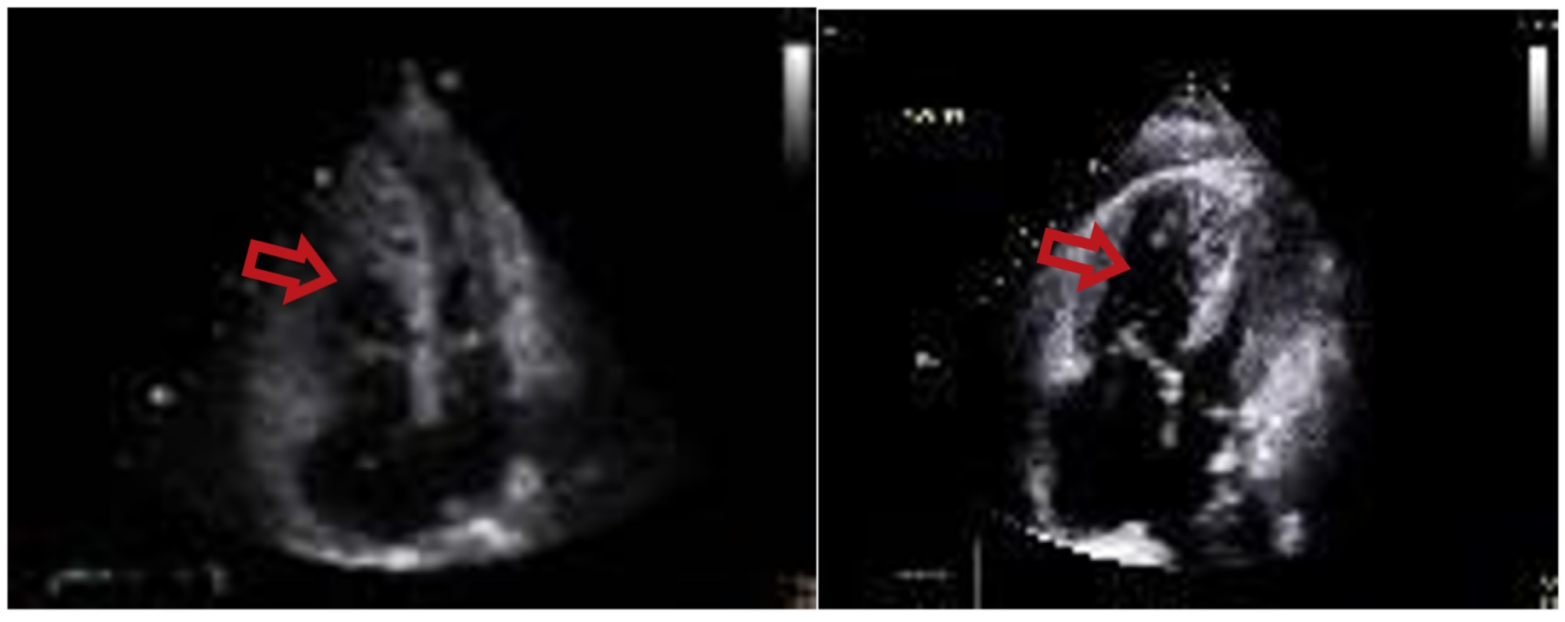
Echocardiogram of a patient with pulmonary hypertension The echocardiogram shows right ventricular dilation (red arrows)
